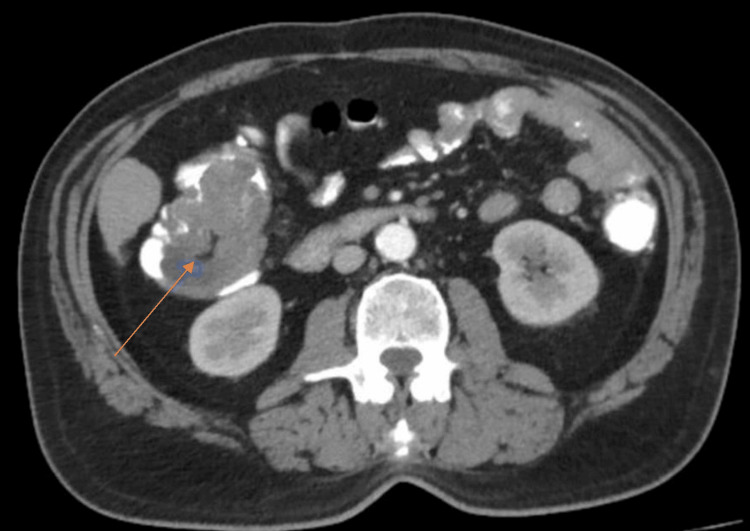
Axial computed tomography scan showing a solid tumor lesion with lobulated contours, located intraluminally within the cecum and part of the ascending colon (arrow)
